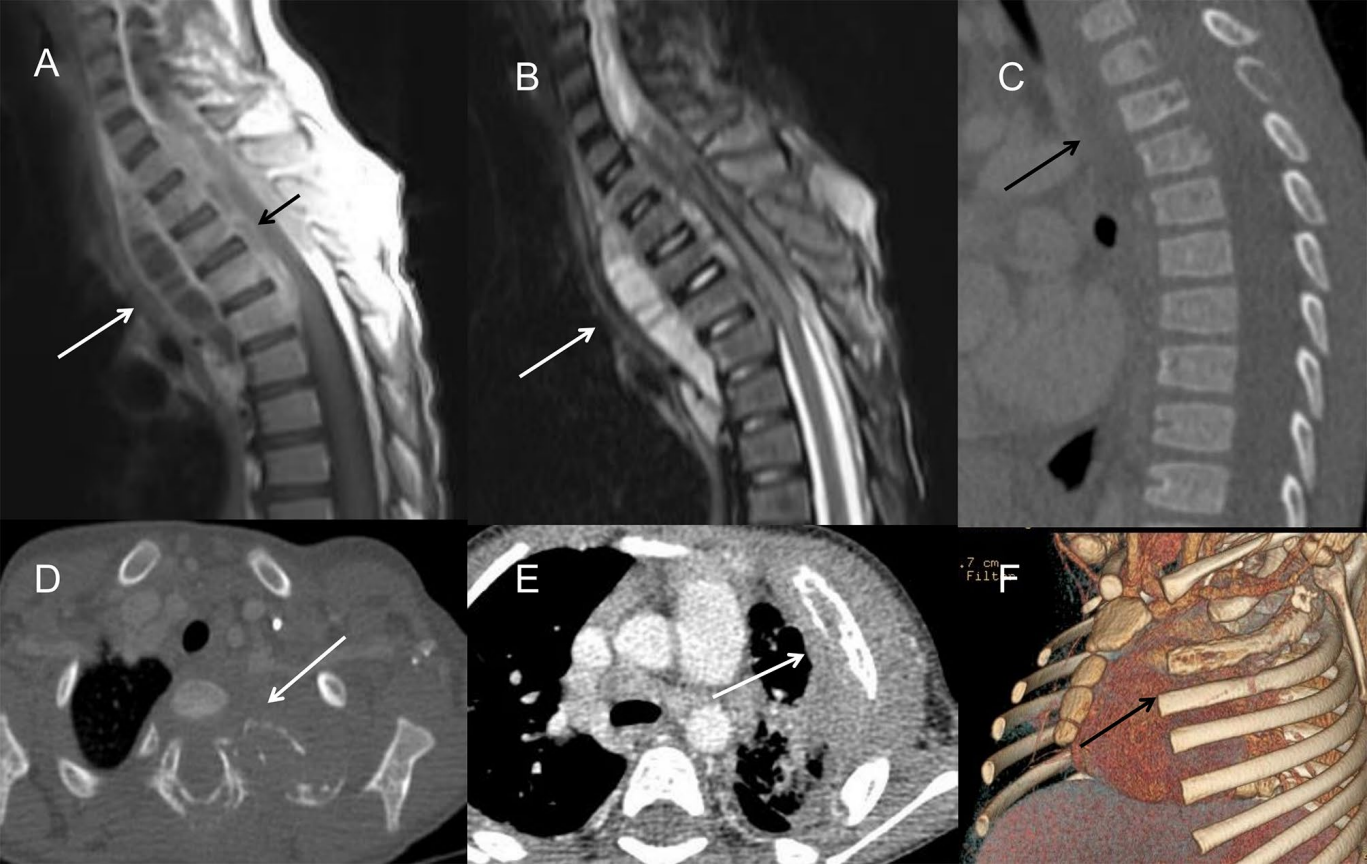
(A–D) A 3-year-old boy with infection of TB. (A) On CT, the 3rd thoracic vertebra was slightly compressed and the 3rd and 4th thoracic vertebra had multiple small areas of osteolysis (long arrow). (B) On T2WI image, paravertebral abscess extending into the spinal canal and compressing the thoracic spinal cord could be found (long arrow). (C) The abscess wall was markedly enhanced on post-contrast T1WI sequence (long arrow). (D) On axial CT image, the osteolysis and expansion of the left first rib and vertebral plate were found (long arrow). (E,F) A 2-year-old boy with fungi infection. On CT images, osteomyelitis of the left 2nd rib and surrounding soft tissue swelling were showed (long arrow). The adjacent lung infection and pleural thickening were also noticed.
